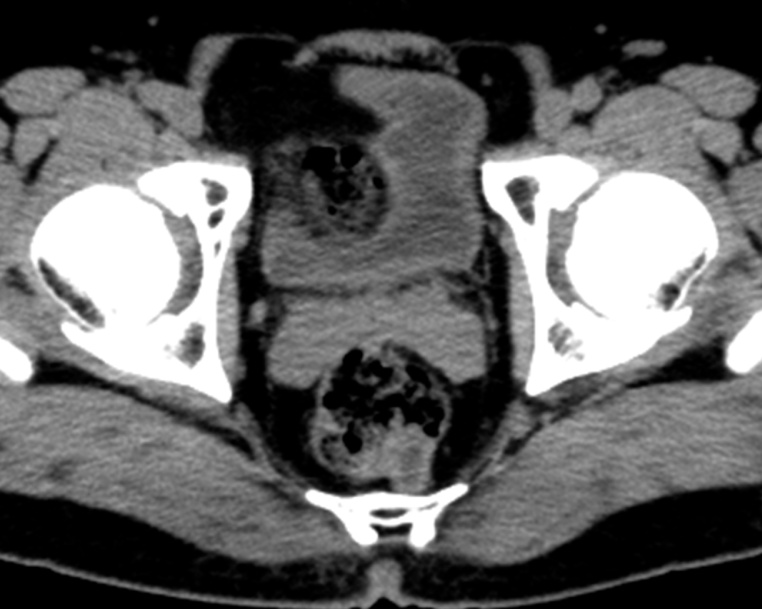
Pelvic CT finding. Pelvic CT showed that a 20-mm low density mass at posterior of lower rectum.
